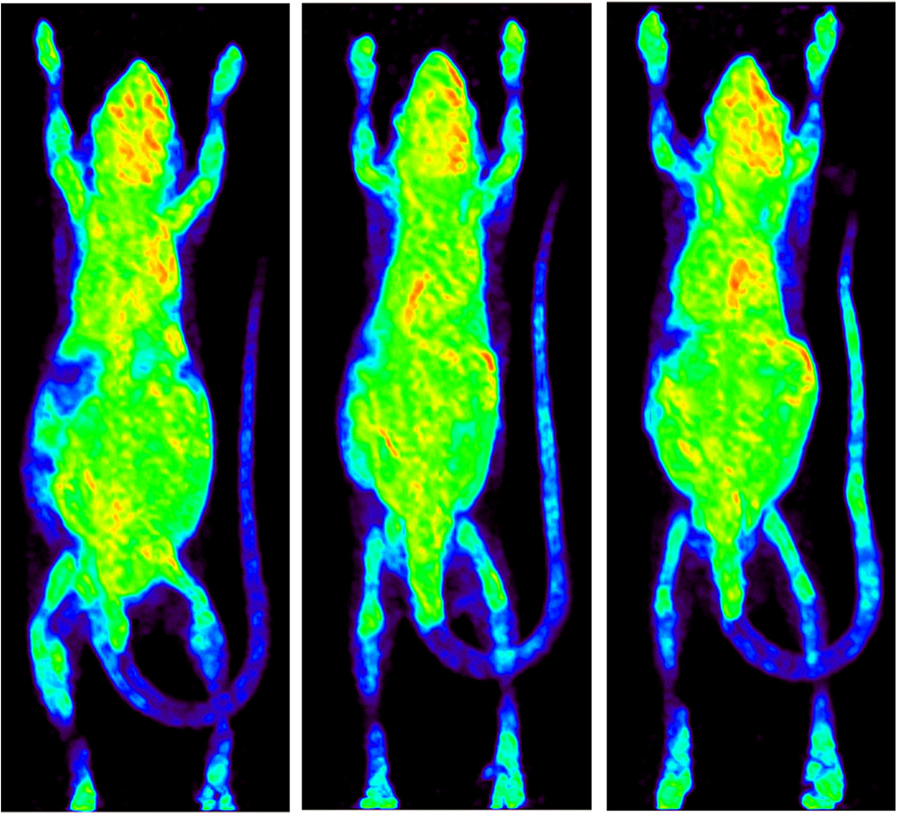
PET image produced from an [^18^F]FDG infusion using the automated dispenser. Images are saturated in order to view the tail. Region of interest analysis on the attenuation corrected images show that the percent of radioactivity in the tail is no more than 2 % of the total radioactivity injected, indicating a good infusion
